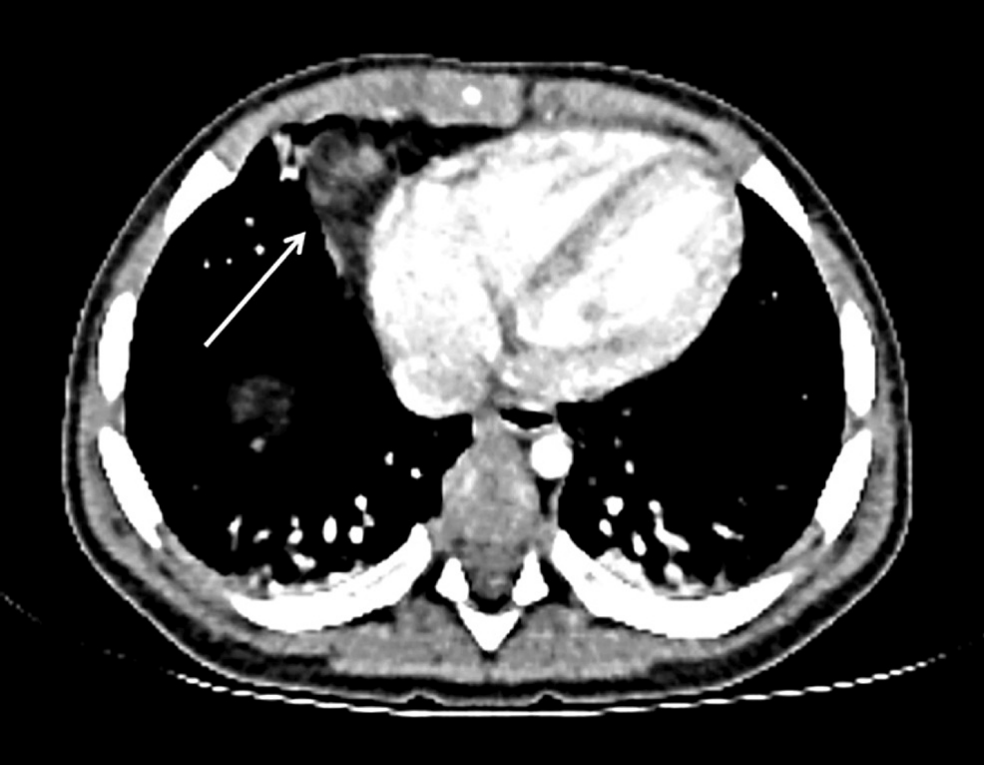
Contrast-enhanced computed tomography showing herniated omentum into the right hemithorax (axial view).
